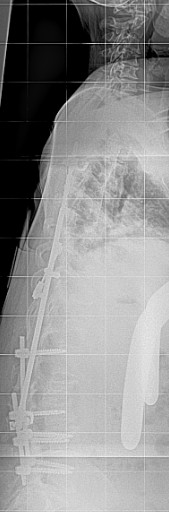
X-ray of the spine in lateral projection after dorsal spondylodesis showing the progressive thoracolumbar kyphosis and looming cutting-out of the pedicle screw in L1
